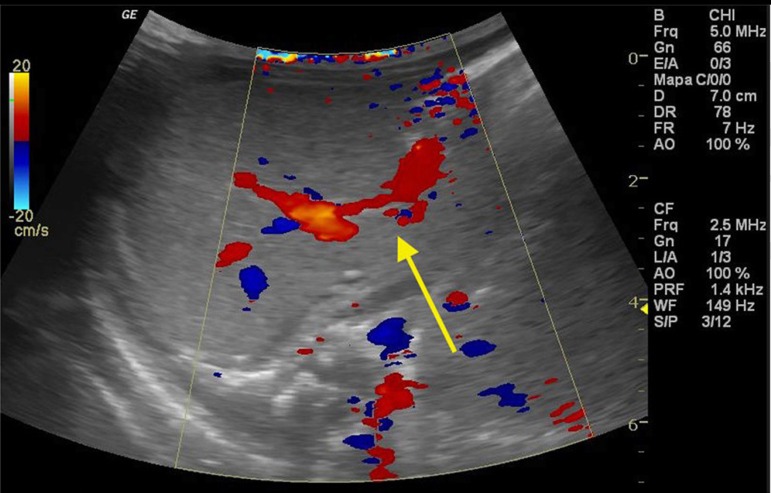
Abdominal ultrasound with Doppler. The arrow indicates the portosystemic shunt.
